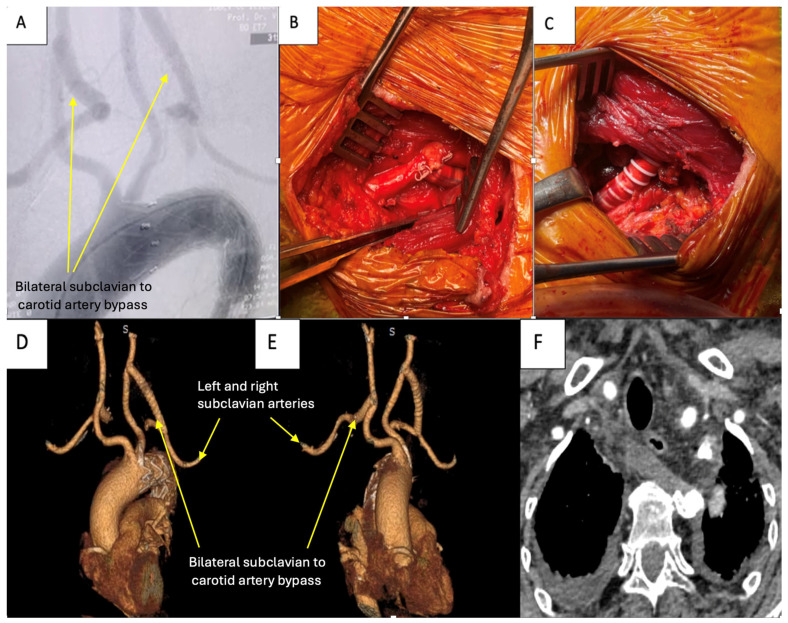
(A) Arteriography showing bilateral subclavian carotid bypass. (B) Intraoperative view of right carotid–subclavian bypass. (C) Intraoperative view of left carotid–subclavian bypass. (D) Postoperative chest CT angiography reconstruction of the aorta and supra-aortic vessels. (E) A 1-month chest CT angiography reconstruction of the aorta and supra-aortic vessels. (F) Postoperative chest CT cross-section showing no compression on the esophagus.
